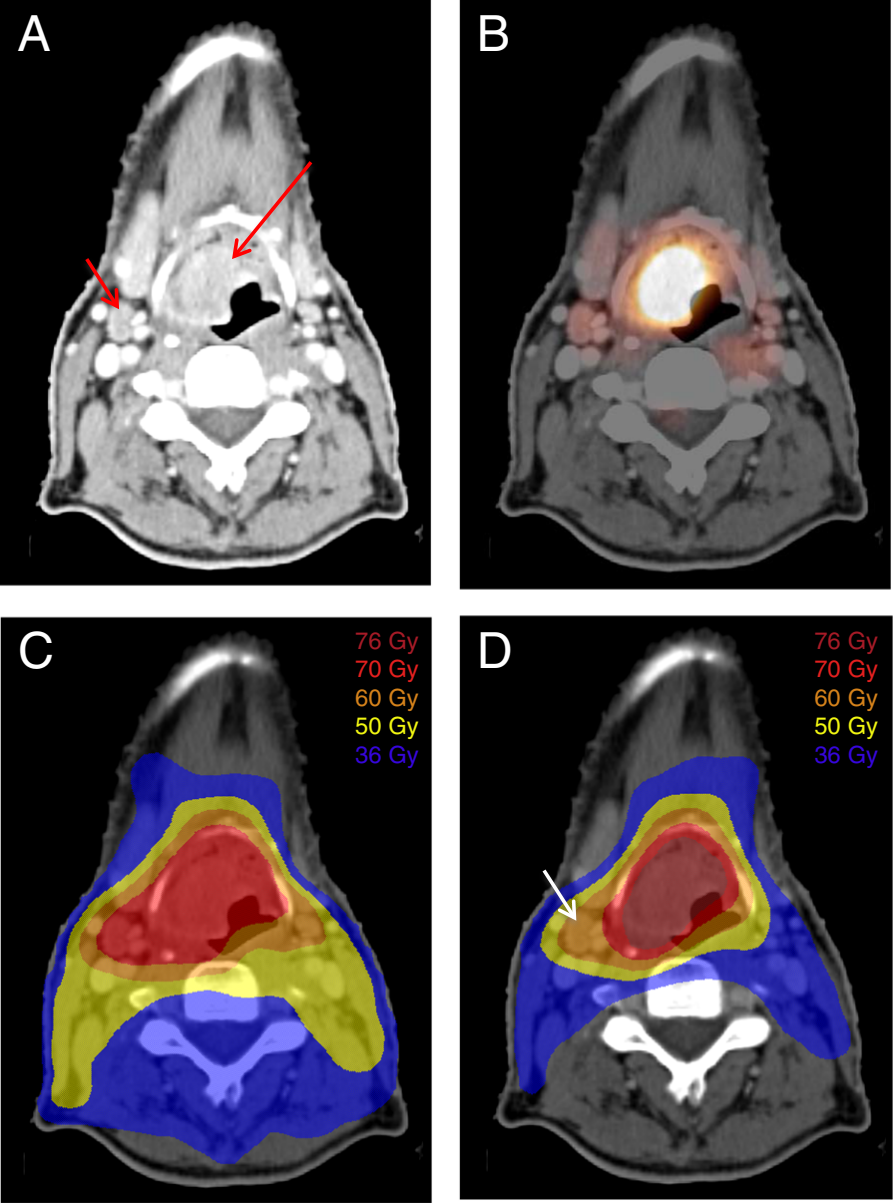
Radiation therapy planning FDG-PET/CT-scan of a patient with an laryngeal squamous cell carcinoma (red arrow) with an intermediate risk lymph node in level 3 right (red arrow) (a + b). Comparison of dose planning conform this study protocol for the control-arm (c) and intervention-arm (d) shows the potential of FDG-PET guided gradient dose prescription with dose reduction to the elective neck in order to better spare organs at risk
